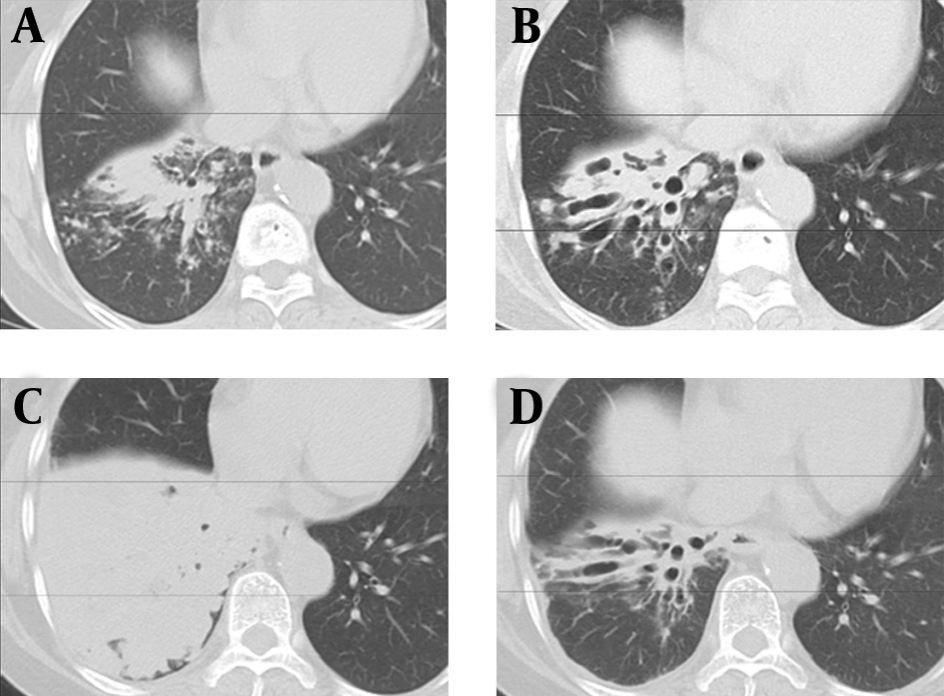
Chest CT Findings Changes Over the Time A) At May 18, 2010 (one month after the first visit), thickened bronchial walls and mucus plugging, causing a “tree-in-bud” pattern, were observed in the right lower lobe. These were considered to be from respiratory tract lesions. B) After four months (September 2), clubbing bronchiectasis was observed. C) After six months (November 18), bronchi of the right lower lobe were further dilated, and mucoid impaction was worsened, while the volume was decreased. D) One month after the TFB removal, clubbing bronchiectasis remained in the right lower lobe, although atelectasis was improved.
